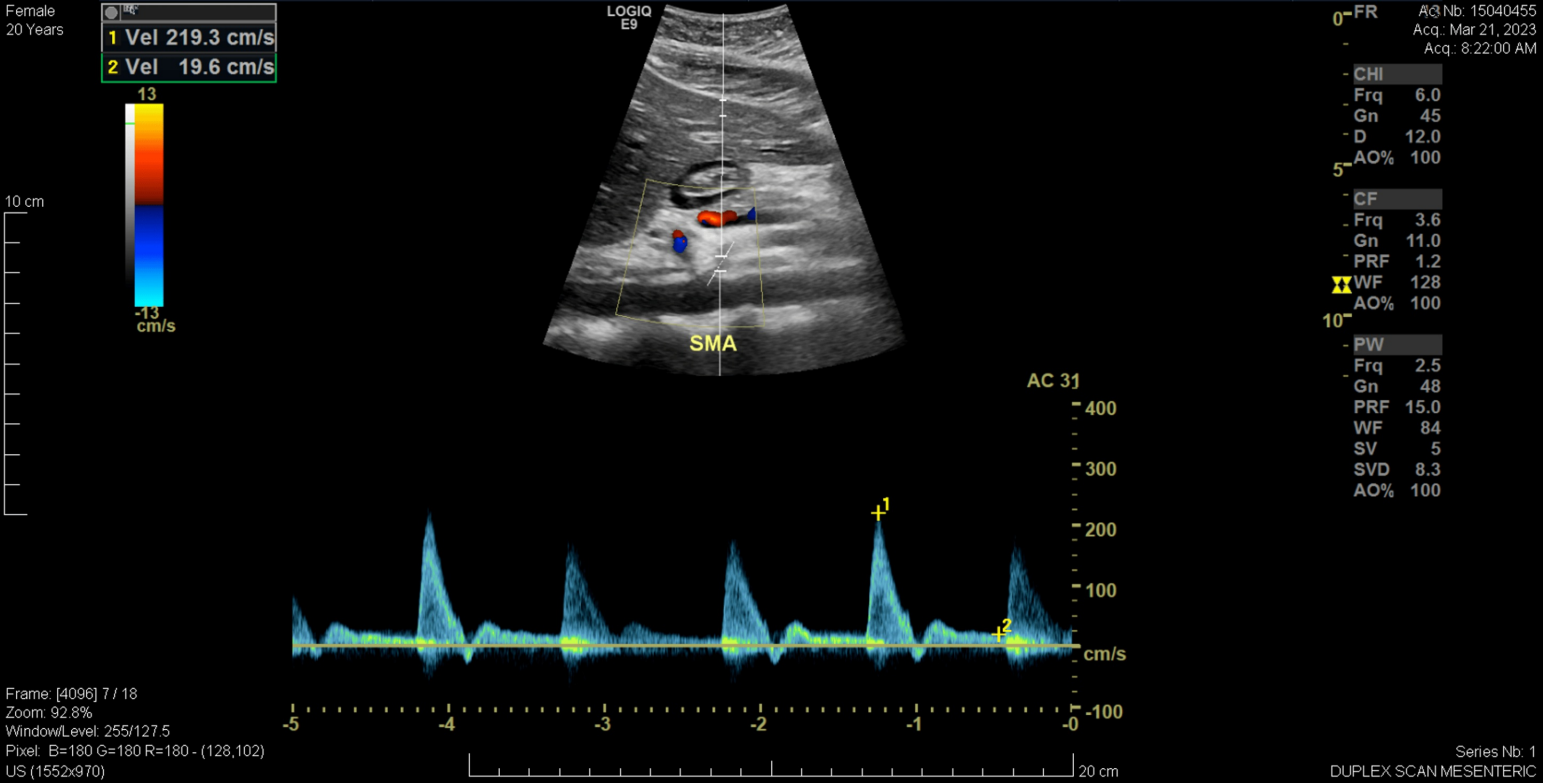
Duplex ultrasound capturing SMA’s peak systolic velocity (219.3 cm/s) at the origin. Elevated velocities were suggestive of superior mesenteric compression. A potential complication of median arcuate ligament syndrome, given the close proximity of the celiac trunk and the low-riding diaphragm, could affect both arteries. SMA: Superior mesenteric artery
